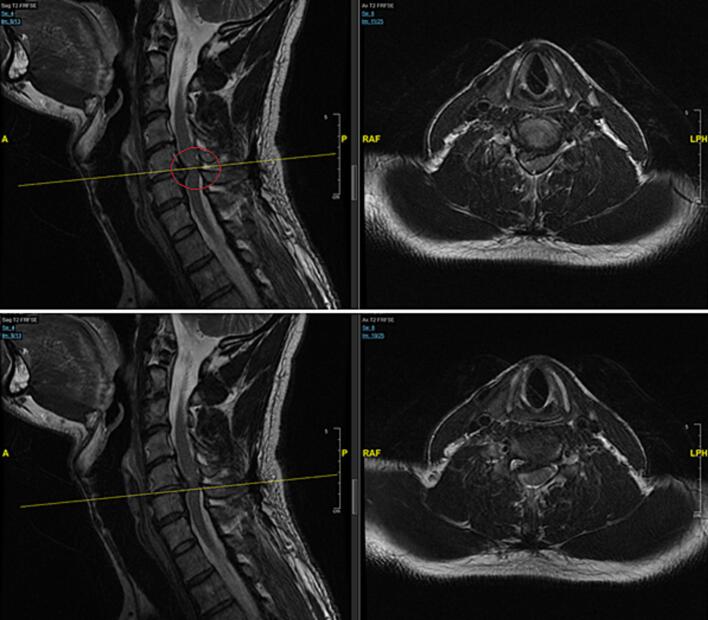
Sagittal and axial T2-weighted MRI images of the cervical spine (3T) demonstrating a large, right paracentral disc extrusion at the C5–C6 level. The disc fragment breaches the posterior longitudinal ligament and exerts significant mass effect on the right anterolateral aspect of the spinal cord, suggestive of intradural extension. The red circle highlights the halo sign. (For interpretation of the references to colour in this figure legend, the reader is referred to the web version of this article.)
